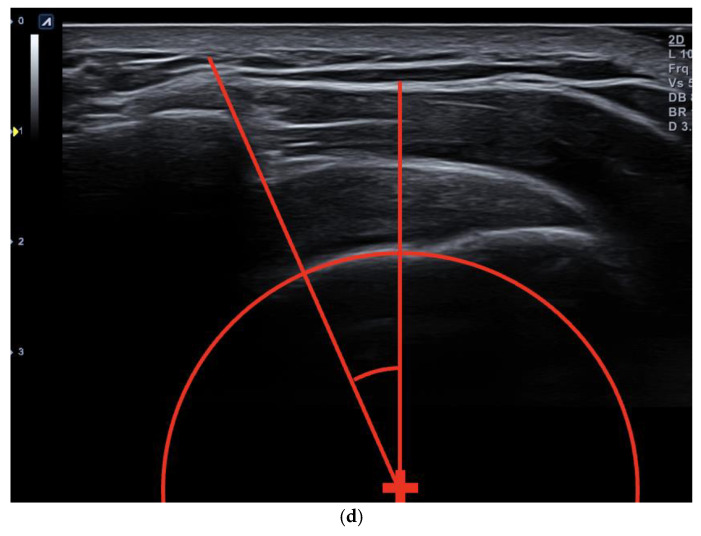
(a) Native ultrasound image of the shoulder joint in superior lateral section based on DEGUM guidelines. (b) Identification of the anatomical structures. (c) Expansion of the depicted circular section of the humeral head. (d) Determination of the mCSA.
